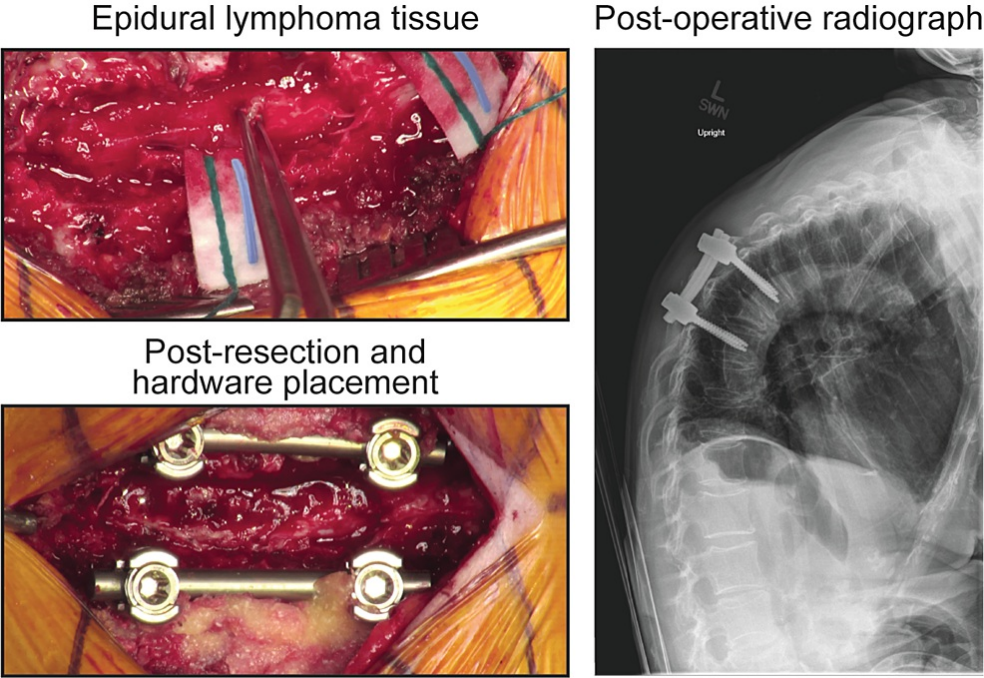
Intraoperative view of epidural lymphoma resection and decompressive laminectomy. T7 – T9 decompressive laminectomy was performed, and epidural lymphoma was observed and resected off the underlying dura. Instrumented fusion was performed to stabilize the spinal column. Post-operative radiograph confirmed stable hardware fixation of T7 – T9.
